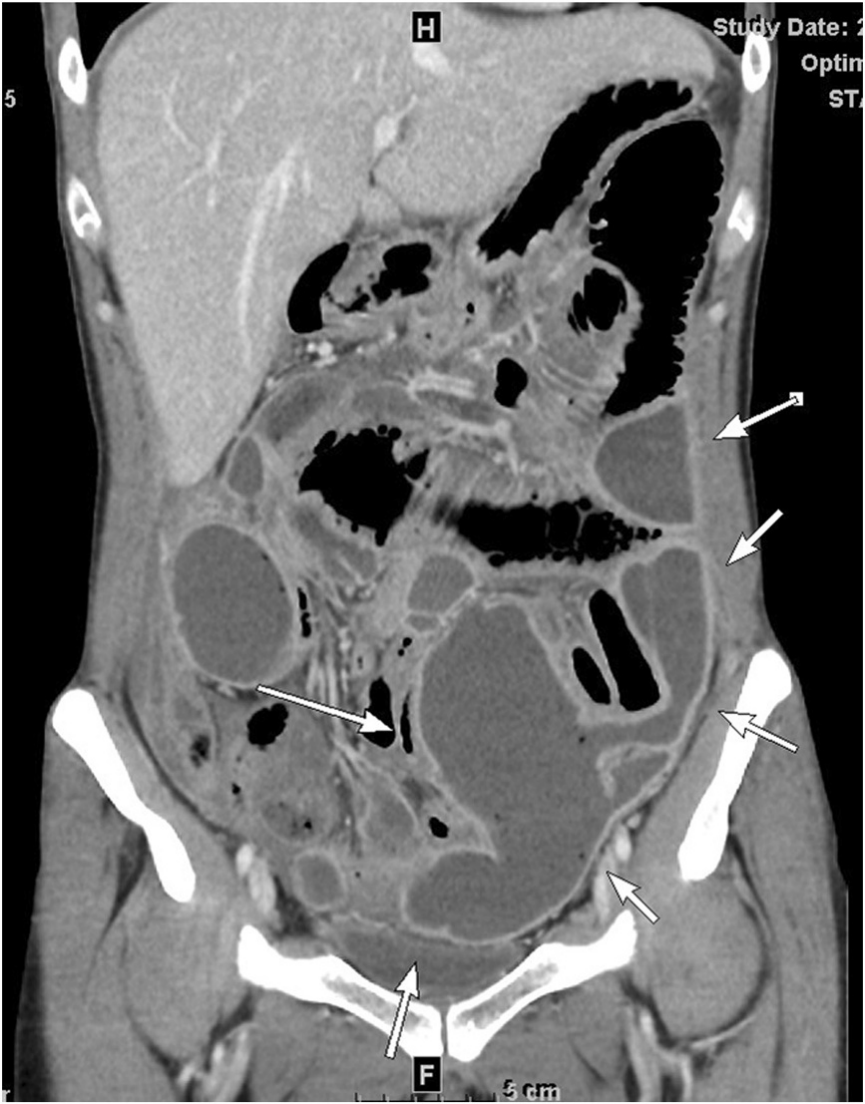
Abdominal computed tomography (CT) scan. An abdominal CT scan showed a massive abscess occupying a significant portion of the intra-abdominal cavity. A well-circumscribed abscess fluid collection with enhanced walls are seen as pointed by the white arrows.
